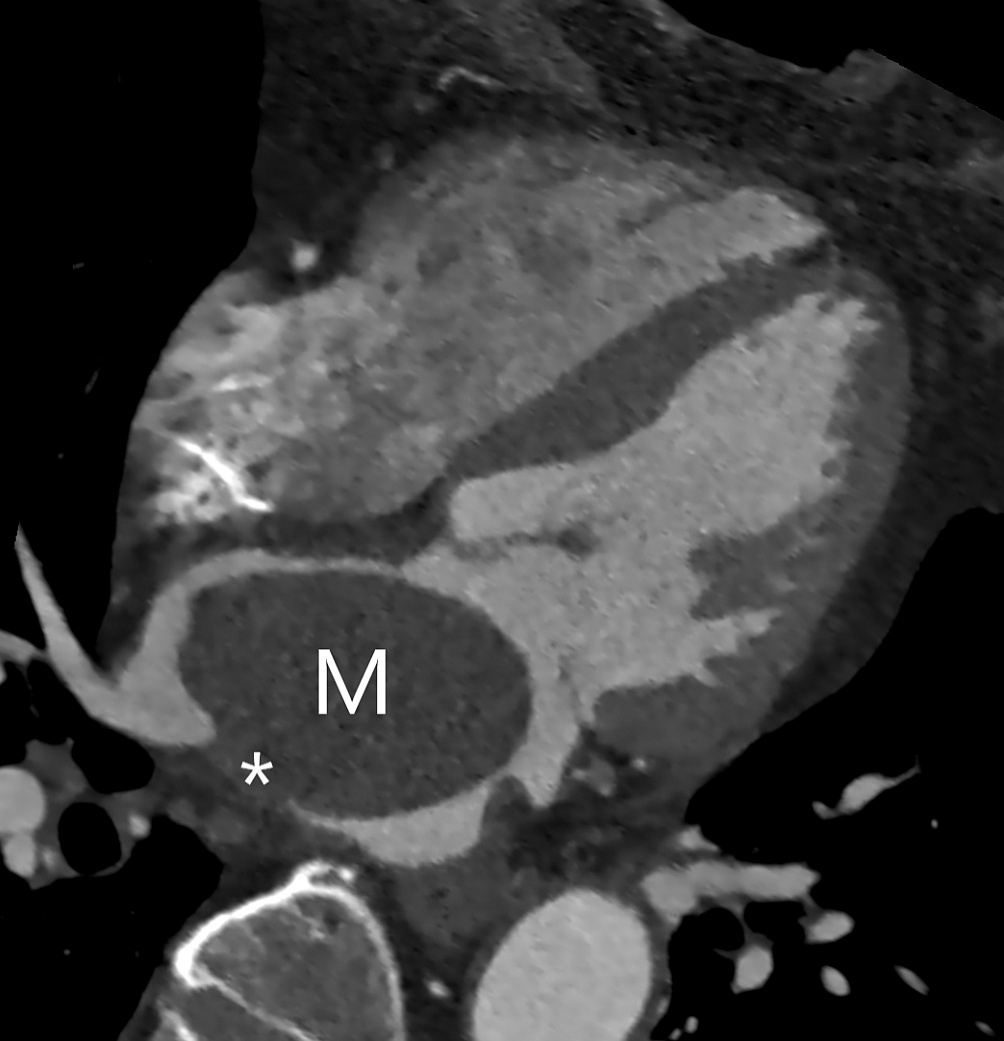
Cardiac CT shows a left atrial myxoma (M) in a less typical
